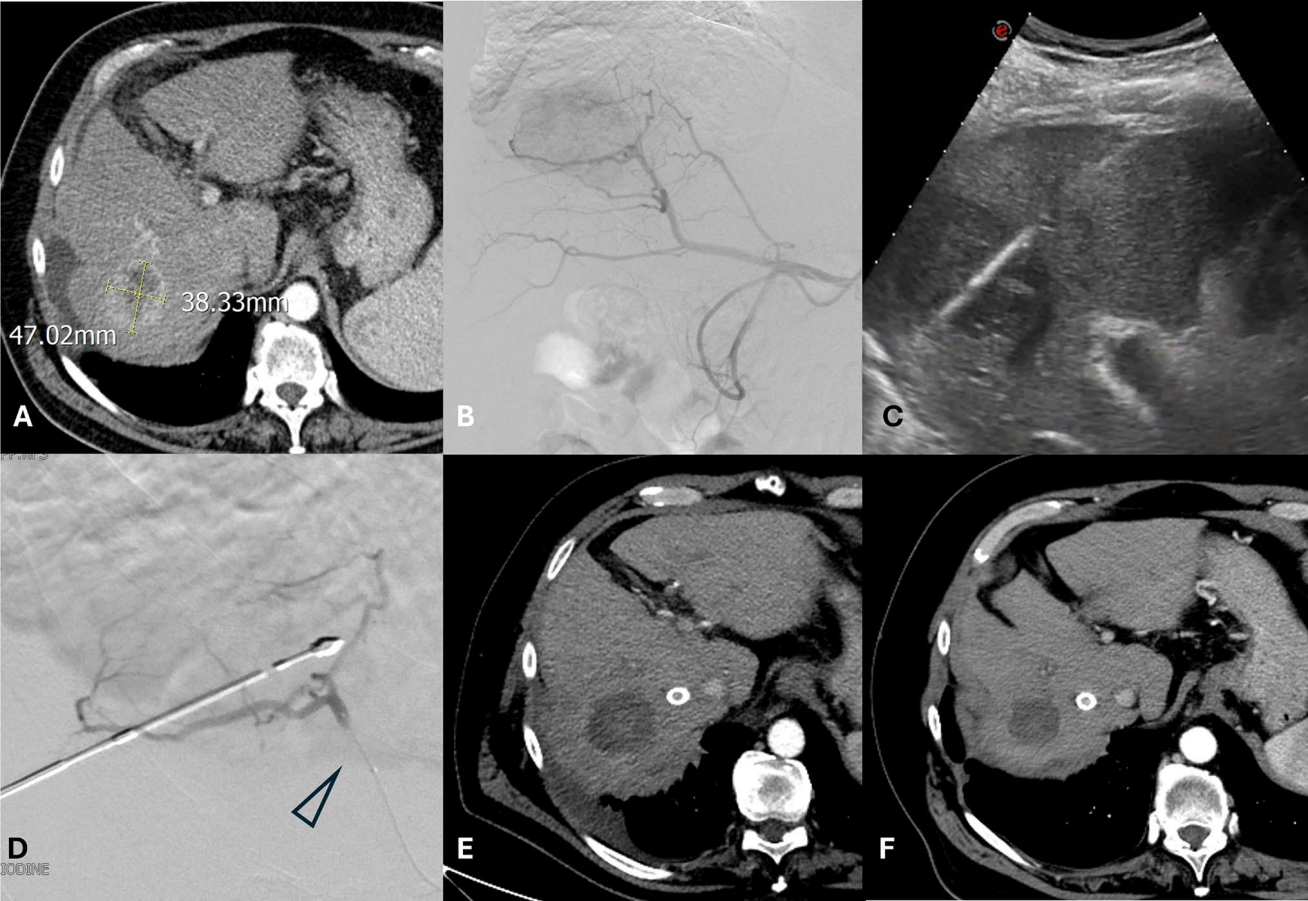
62 years old man with alcoholic cirrhosis in OLT waiting list, with portal and mesenteric vein thrombosis, for a 47-mm HCC located in segment VIII, partially necrotic and hypervascular at the CT arterial phase (a). Percutaneous thermal segmentectomy was performed as bridging two weeks before TIPS creation. Digital subtraction arteriography (b) confirmed the hypervascular lesion; MW antenna was positioned under US guidance (c) and ablation performed after micro-ballon catheter inflation (arrowhead, d). One month CT follow-up, performed after TIPS placement, showed Complete response of the lesion, sustained up to 15 months (f)
